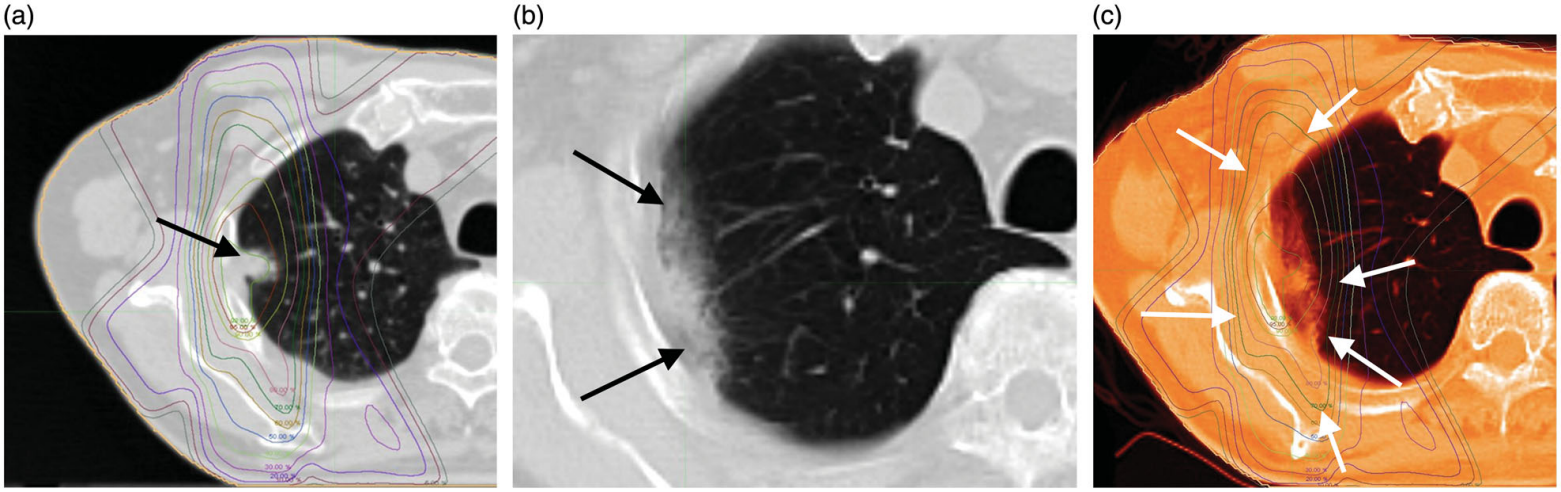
(a) An 84-year-old man with a 14-mm-diameter adenocarcinoma in the right upper lobe S2 of the lung (black arrow). (b) This shadow appeared as a "Scar-like pattern" in late phase computed tomography (CT) imaging at 559 days after radiotherapy (black arrows). (c) Treatment planning and acute CT imaging were fused with MIM Maestro. The shadow of RILI was found in the 70% dose region (white arrows).
